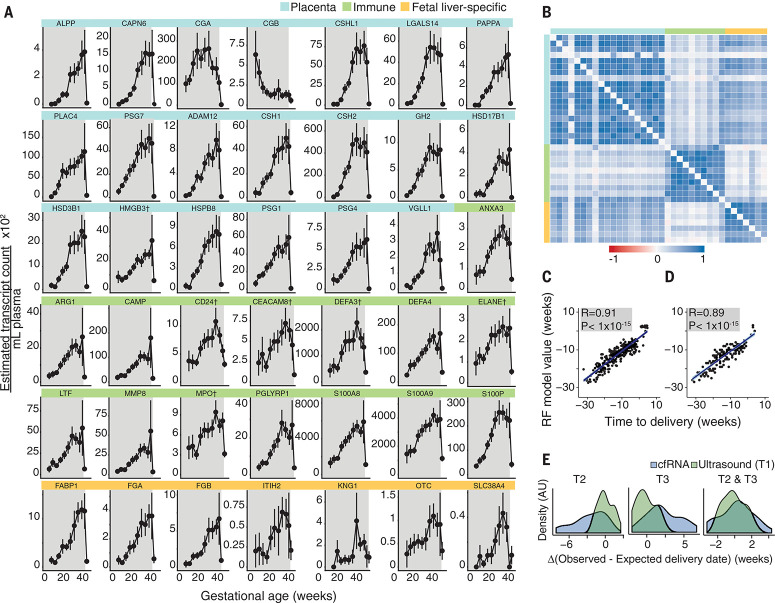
Application of cfRNA measurements to predict gestational age. (A) For each gene, cfRNA transcript count measurements are shown over the course of gestation. Each point represents the mean cfRNA value ± SEM for either 31 women or 21 women (the latter denoted by †). The antepartum period is highlighted in gray. Placental, immune, and fetal liver–specific genes are highlighted in blue, green, and orange, respectively. (B) Heat map of the Pearson correlation coefficient for each gene pair shows that placental, immune, and fetal liver–specific cfRNA [same group colors as (A)] measurements are highly correlated with each other [median Pearson correlation *r* = 0.79 (placenta), 0.79 (immune), 0.74 (fetal liver); *P* < 10^−14^]. Placental and fetal liver–specific genes also show a weak degree of cross-correlation (*r* = 0.47, *P* < 10^−15^). Gene order matches order shown in (A), omitting genes denoted by † in (A). (C) Cross-validated random forest (RF) model predicts time to delivery from sampling time point (*R* = 0.91, *P* < 10^−15^, *n* = 21) for training cohort. (D) Cross-validated random forest model predicts time to delivery from sampling time point (*R* = 0.89, *P* < 10^−15^, *n* = 10) for validation cohort. (E) Distribution of difference in weeks between observed and predicted gestational age at delivery using cfRNA measurements from the second (T2), third (T3), or both (T2 & T3) trimesters (left to right) versus using ultrasound measurements from the first trimester (T1). AU, arbitrary units.
